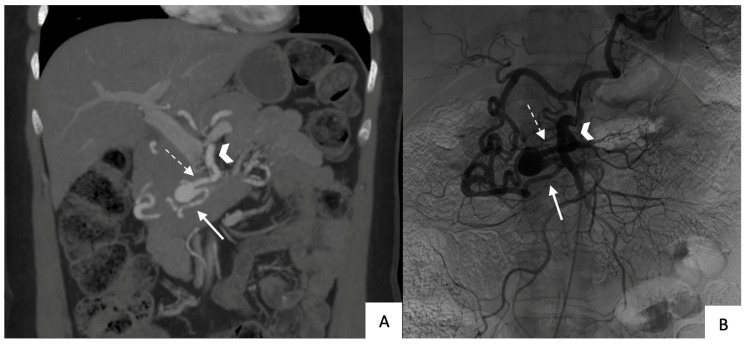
Patient with an unruptured pancreaticoduodenal artery aneurysm: (A) Computed tomography in a coronal view with maximum-intensity-projection reformatting showing the aneurysm (arrowhead: superior mesenteric artery; dotted arrow: afferent branch; solid arrow: efferent branch); (B) superior mesenteric artery angiogram in the same patient (same annotation).
